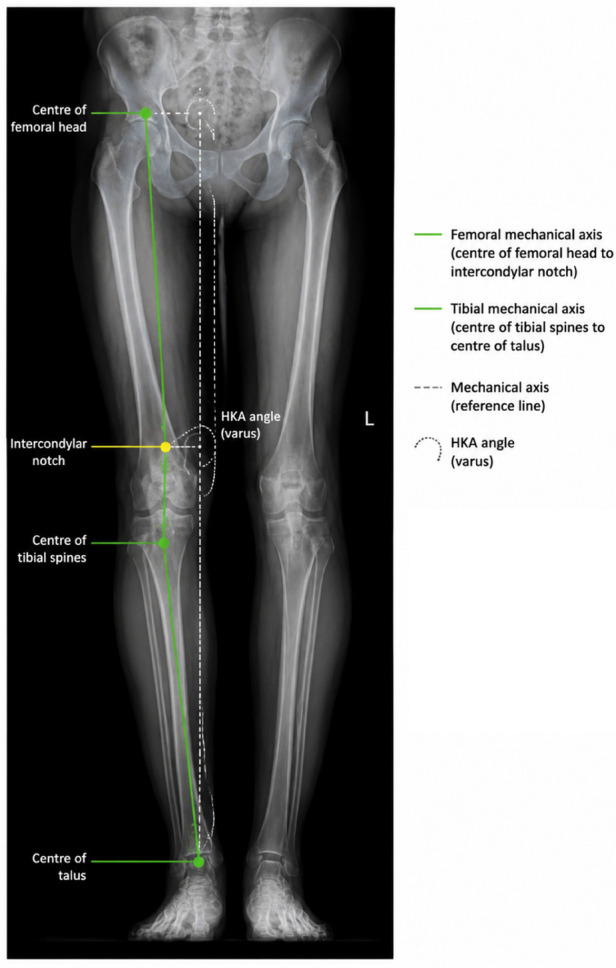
Preoperative full-length weight-bearing anteroposterior radiograph of the lower extremities used to measure the mechanical axis, hip–knee–ankle (HKA) angle, and preoperative varus deformity. The femoral mechanical axis was defined by the line from the centre of the femoral head to the intercondylar notch, and the tibial mechanical axis by the line from the centre of the tibial spines to the centre of the talus.
